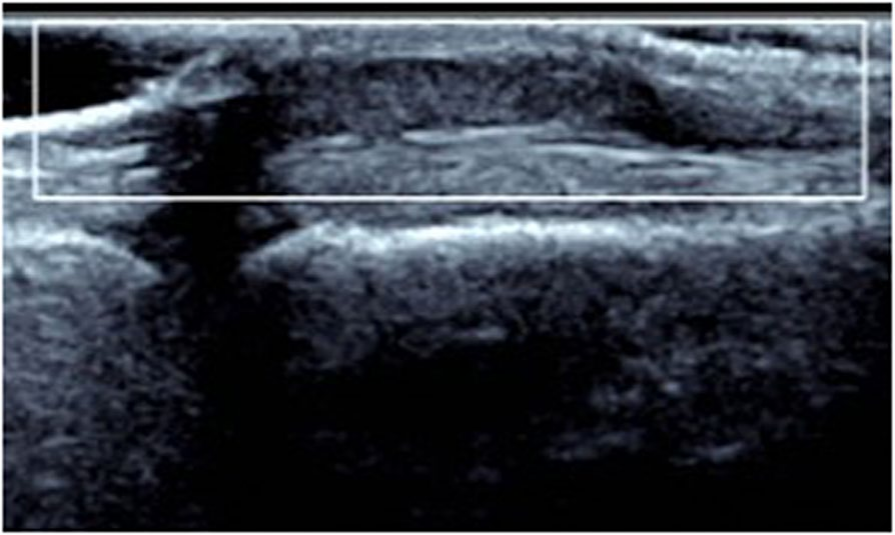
A 29-year-old man with a painless mass on the flexor surface of the thumb. Sonography shows a homogeneous hypoechoic nodule with no blood flow (Class 0)
